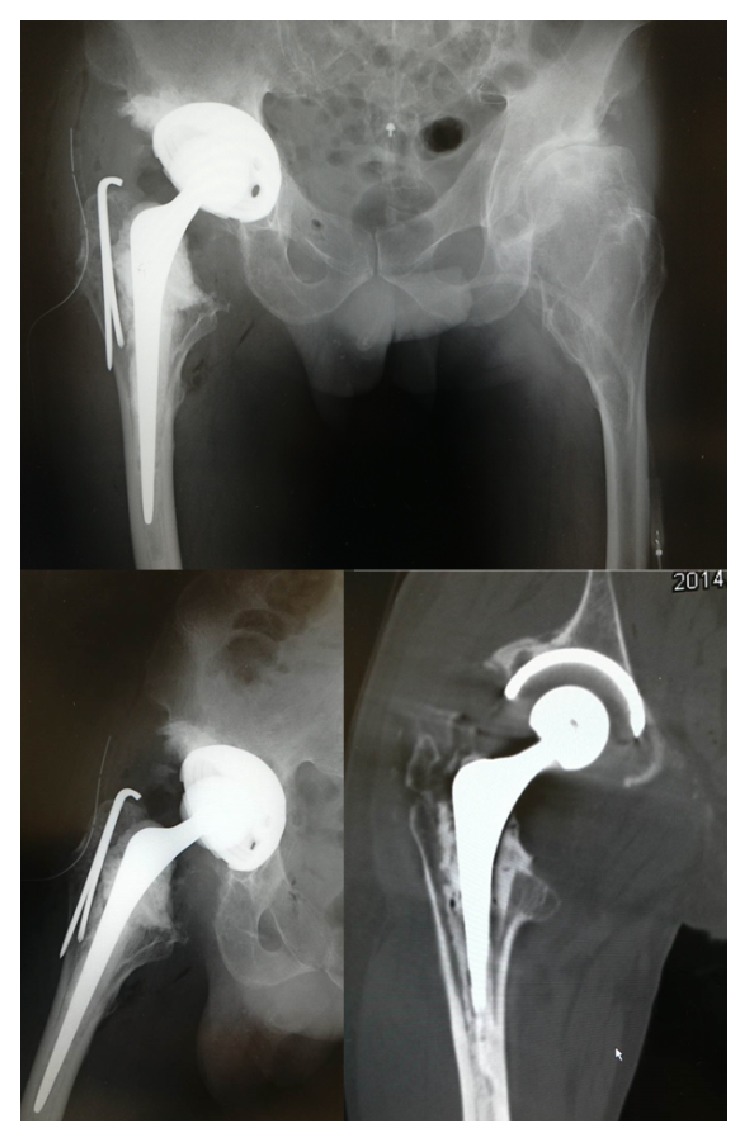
Postoperative radiographs and multidirectional computed tomography imaging showing that the implant setting was unproblematic, and the exostosis at femoral neck had been removed.
